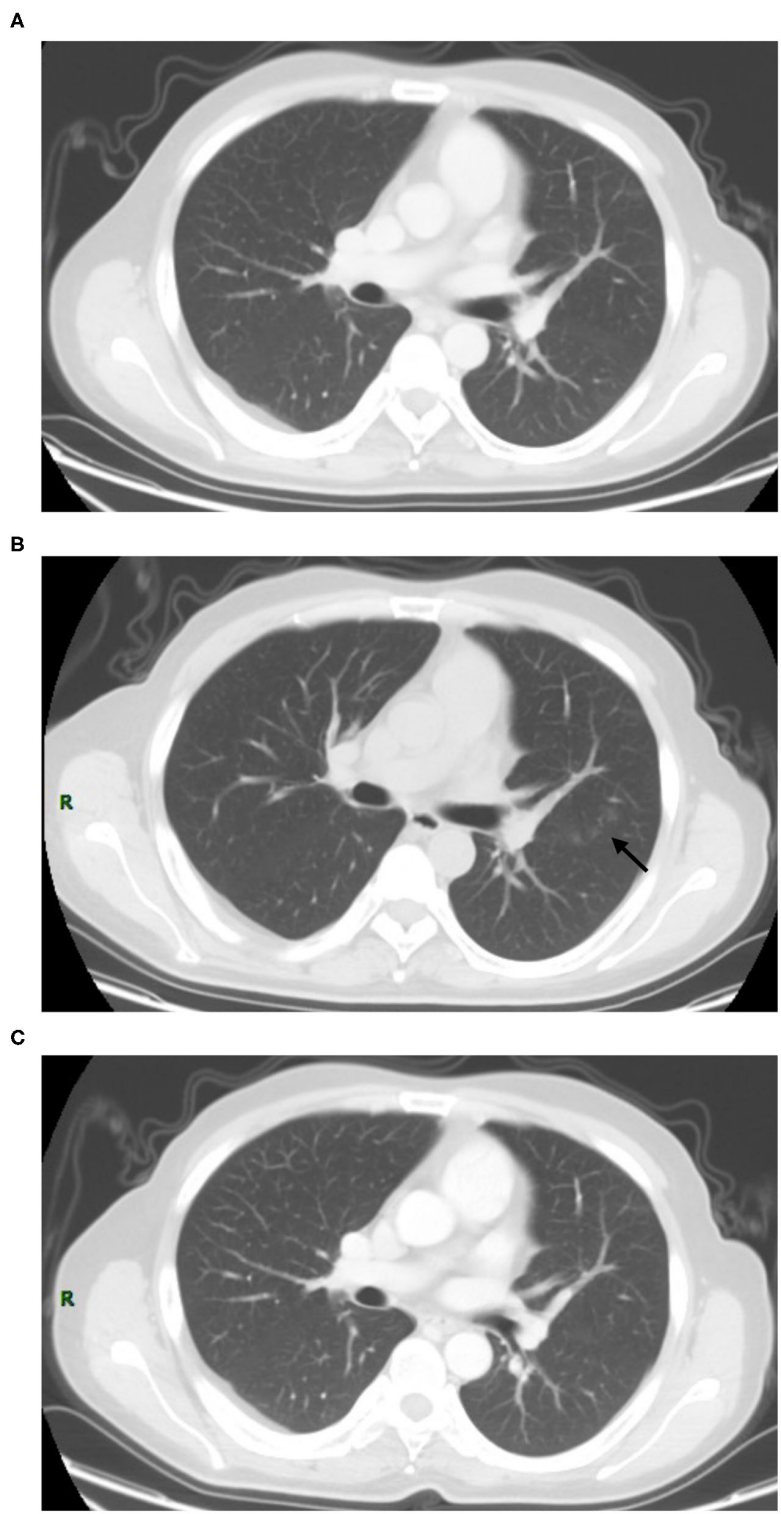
Thirty-seven-year-old male with nasopharynx cancer. Patchy ground glass opacities affecting the left upper lobe had been newly seen (B) (arrow) when compared to the prior CT which performed at 2 months ago (A). The short-term CT scan (C) showed the lesion had been completely resolved.
